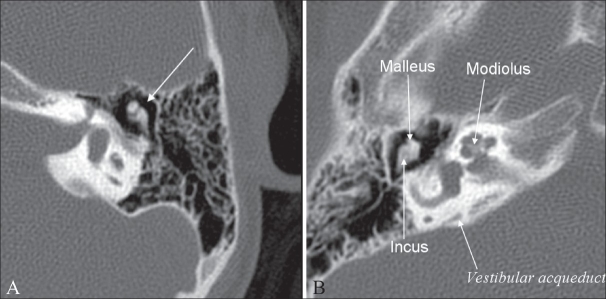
Ice-cream cone sign. Axial high-resolution CT scans of the temporal bone (A-left and B-right) show the ice-cream cone appearance of the incus and malleolus (long arrow in A). The different structures of the middle and inner ear are shown in B
